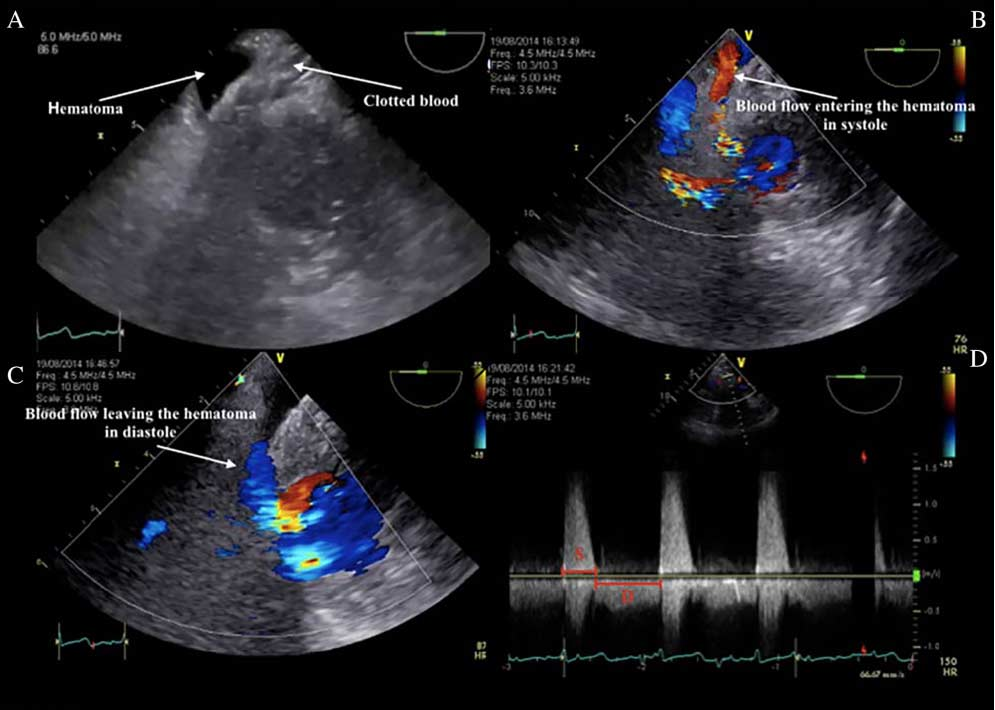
(A) Transgastric mid-papillary views demonstrating a hypoechogenic density, i.e., pericardial haematoma adjacent to clotted blood. (B) CF Doppler demonstrating blood flow entering the haematoma. (C) CF Doppler demonstrating blood flow leaving the haematoma. (D) CW Doppler showing the timing of blood flow through the LV rupture relative to the cardiac cycle. Blood blow is entering the haematoma in systole and leaving the haematoma in diastole. LV, left ventricle; CF, colour-flow; CW, continuous wave; S, systole; D, diastole.
